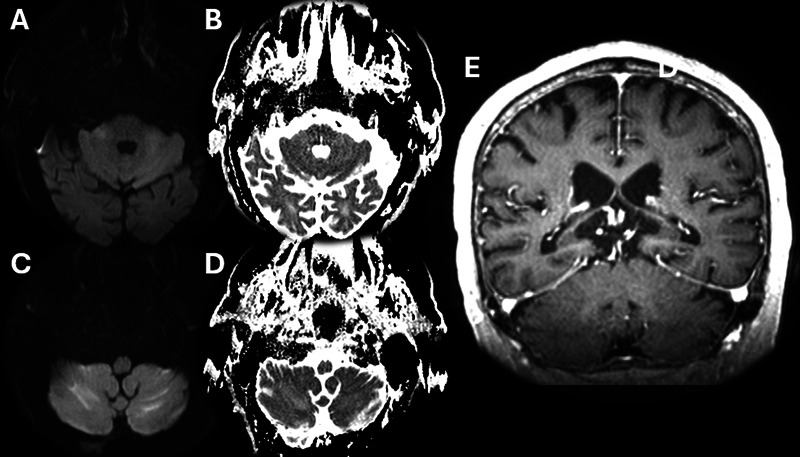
Brain MRI scan, axial diffusion-weighted imaging (
